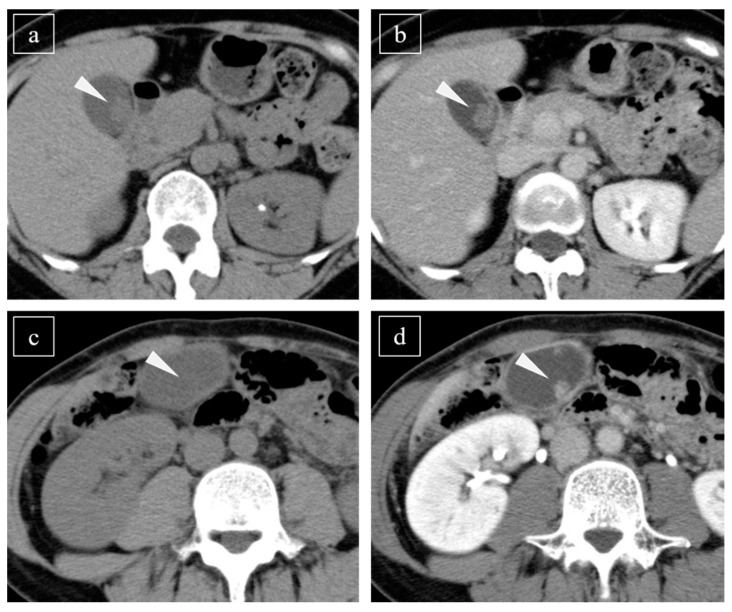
Typical cases of gallbladder polyps detected on plain computed tomography (CT). A pedunculated mass in the gallbladder is recognized using plain CT ((a), arrowhead) and contrast-enhanced CT ((b), arrowhead); this was determined to be a gallbladder carcinoma. For a gallbladder cholesterol polyp, no lesion is seen in the gallbladder using plain CT ((c), arrowhead); however, an enhanced pedunculated mass is detected using contrast-enhanced CT ((d), arrowhead).
